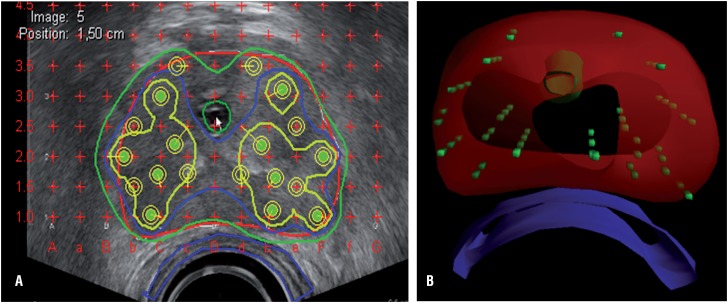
Left: Image of intra-operative planning on transrectal ultrasound. The colored lines mark the different areas where the radiation dose is similar. Urethra and rectum are marked to keep the radiation dose as low as possible in these regions. Right: 3D reconstruction of the seeds after implantation.
